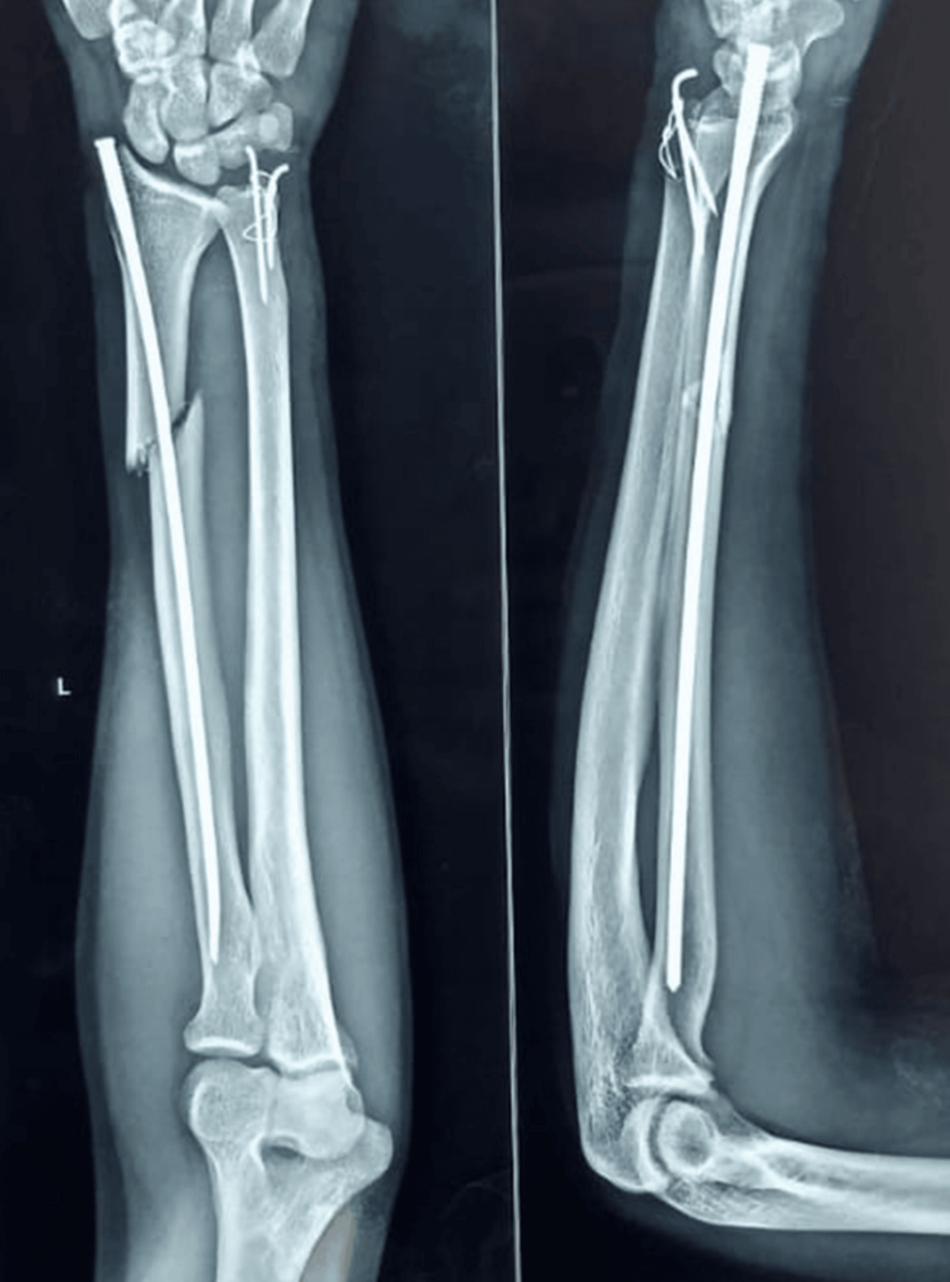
Immediate post-op X-ray of fracture shaft radius managed with screw nail osteosynthesis with tension band wiring (TBW) for ulnar styloid.
